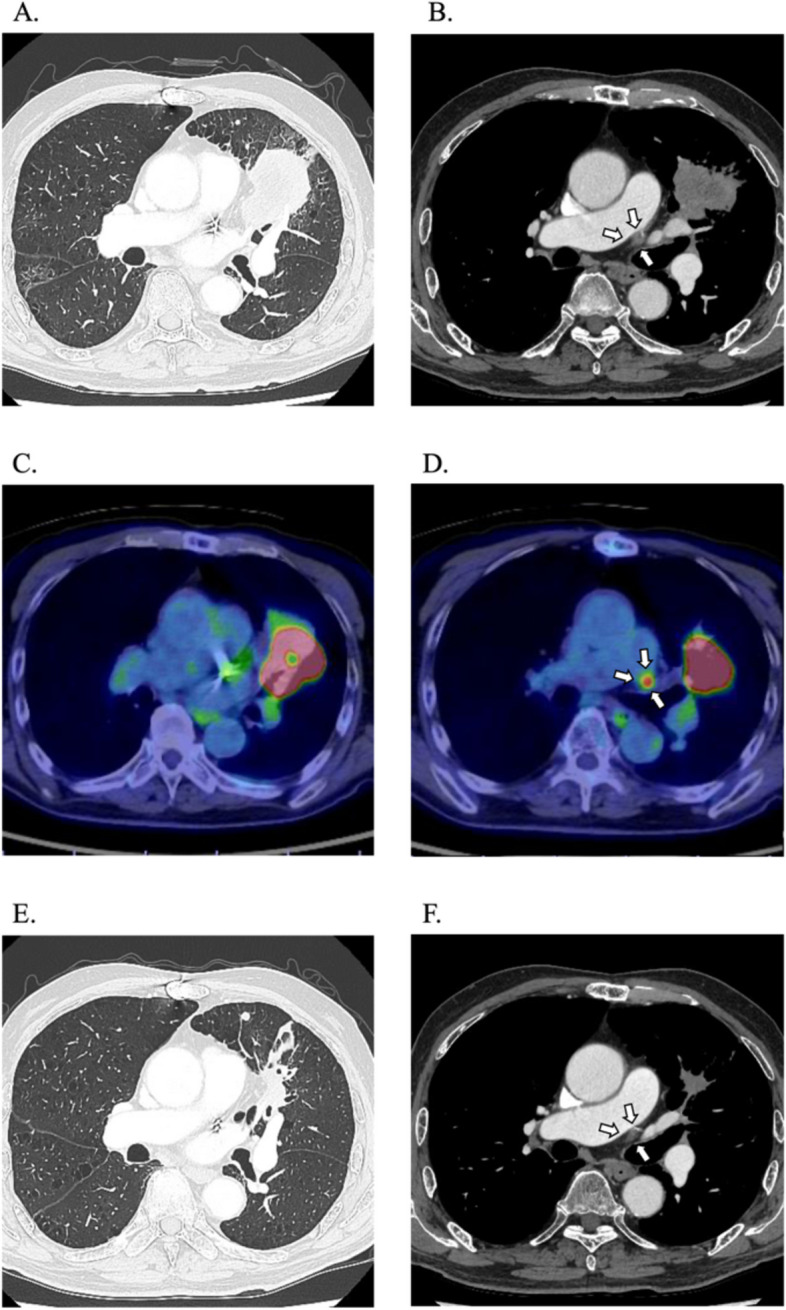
Computed tomography (CT) scan of primary lesion (A) and left hilar lymph node (B) before neoadjuvant nivolumab combination chemotherapy. Positron-emission tomography/CT of primary lesion (C) and left hilar lymph node (D) before neoadjuvant nivolumab combination chemotherapy. CT scan of primary lesion (E) and left hilar lymph node (F) after one cycle of neoadjuvant combination chemotherapy
